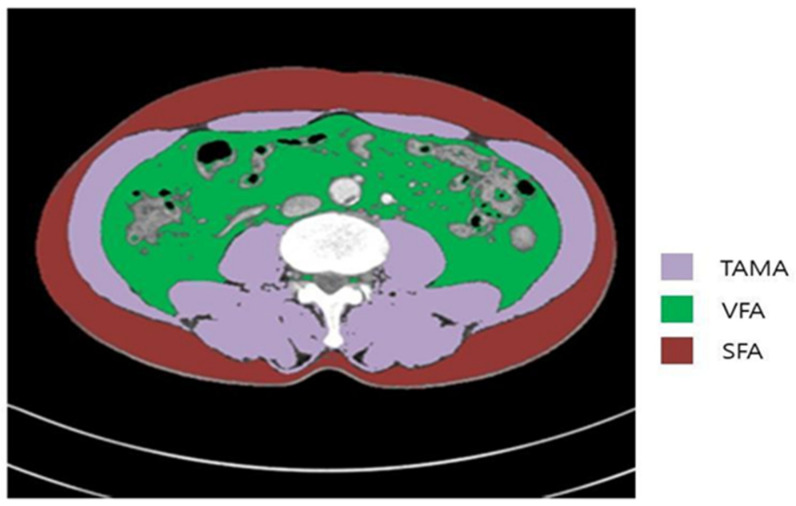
Body morphometric evaluations of abdominal fat and muscle areas. At the level of the inferior endplate of the L3 vertebra, an axial CT image was segmented into the total abdominal muscle area (TAMA), visceral fat area (VFA), and superficial fat area (SFA).
